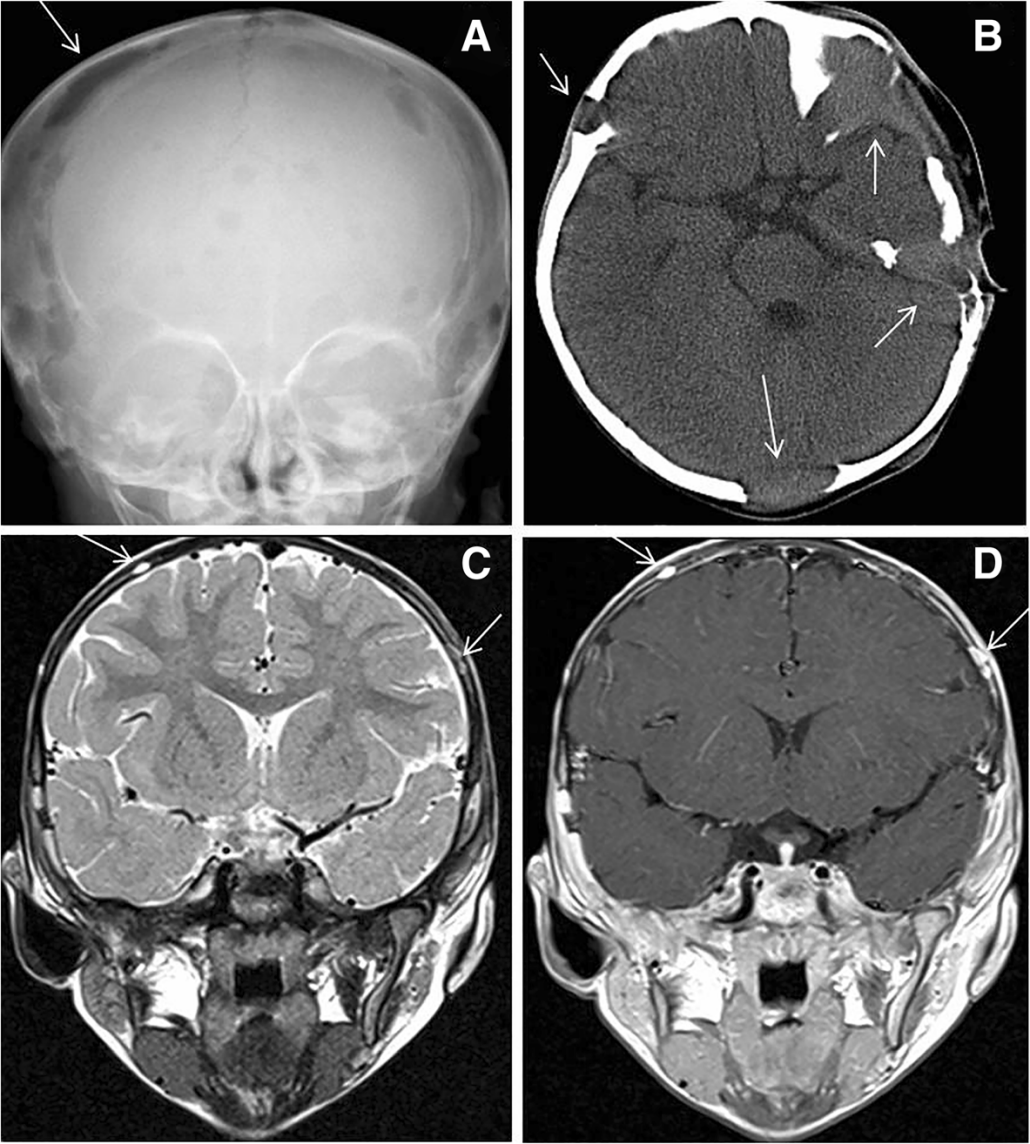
Three-year-old female presenting with multiple tumors of the skull. a In the plain radiography, map like bone destruction (arrow). b Axial post-contrast computed tomography (CT) in bone window showed an aggressive lytic lesion with no peripheral sclerosis (arrow). c T2-weighted high signal is demonstrated in bilateral parietal bone (arrow). d Axial enhanced-contrast T1-weighted MRI showed obvious enhancement (arrow)
